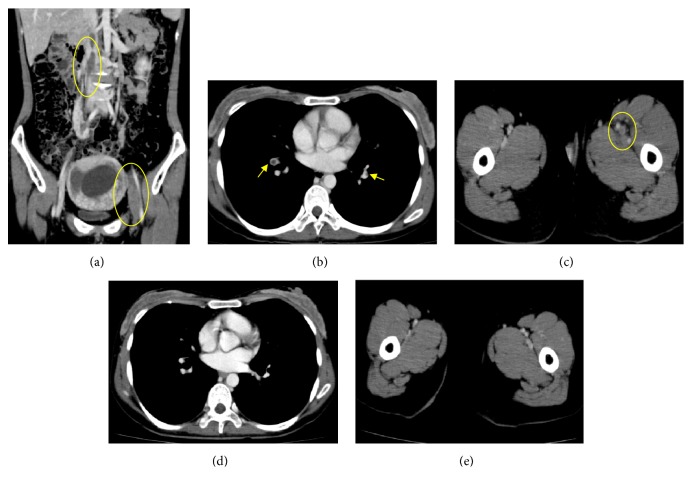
Development of severe VTE, and its disappearance after treatment at patient's fourth pregnancy. (a~c) Contrast CT image taken at the admission day. (a) Coronal plane, large thrombosis exists in inferior vena cava and femoral vein. (b) Transverse plane at the level of trunk of pulmonary artery. (c) Transverse plane at the level of femoral vein. Yellow circle and arrow indicate the location of venous thrombosis. (d, e) Contrast CT image taken at the start of oral administration of edoxaban. (d) Transverse plane at the level of trunk of pulmonary artery. (e) Transverse plane at the level of femoral vein.
